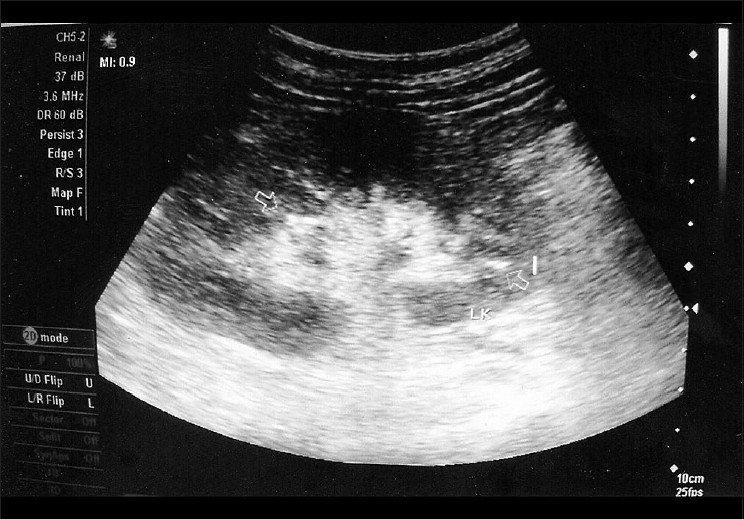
Ultrasound image of the left kidney showing echogenic foci in the renal sinus region (marked with open arrow)
